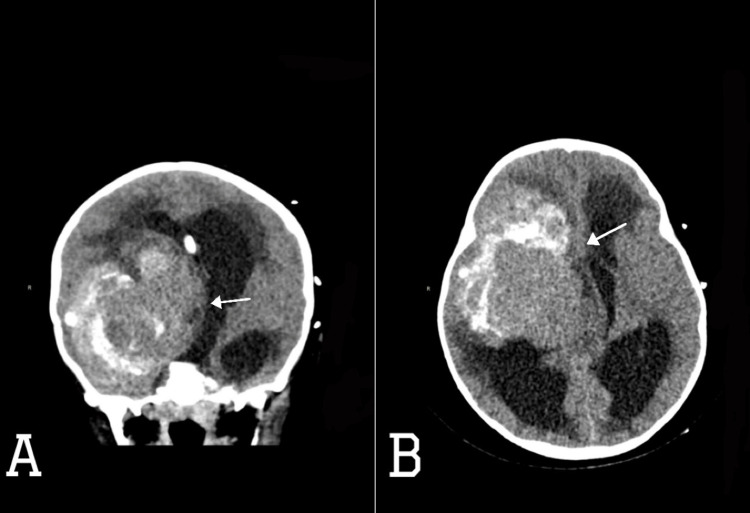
CT scan of the brain (A) axial and (B) coronal views showing right frontotemporal mixed iso-dense to hyper-dense mass measuring 6.7 x 7.5 x 7.1 cm in transverse x anteroposterior x craniocaudal diameters (white filled arrows), causing significant mass effect on and displacement of the right lateral ventricle frontal and temporal horns, third ventricle, right basal ganglia, right cerebral peduncle with 0.9 cm leftward midline shift, and active supratentorial hydrocephalus.
